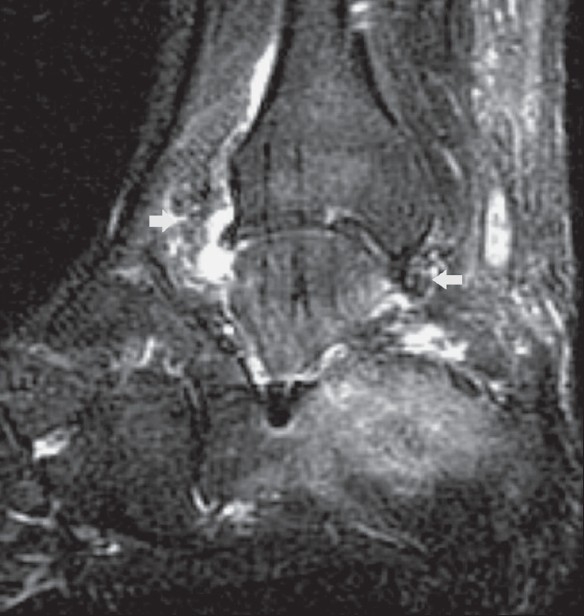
Tuberculous arthritis of the right ankle joint. Sagittal, fatsuppressed T2W MRI shows hyperintense joint effusion within the tibiotalar and subtalar joints. The thickened synovium is hypointense (white arrows). Note also bone marrow edema in the distal tibia, talus and calcaneum
